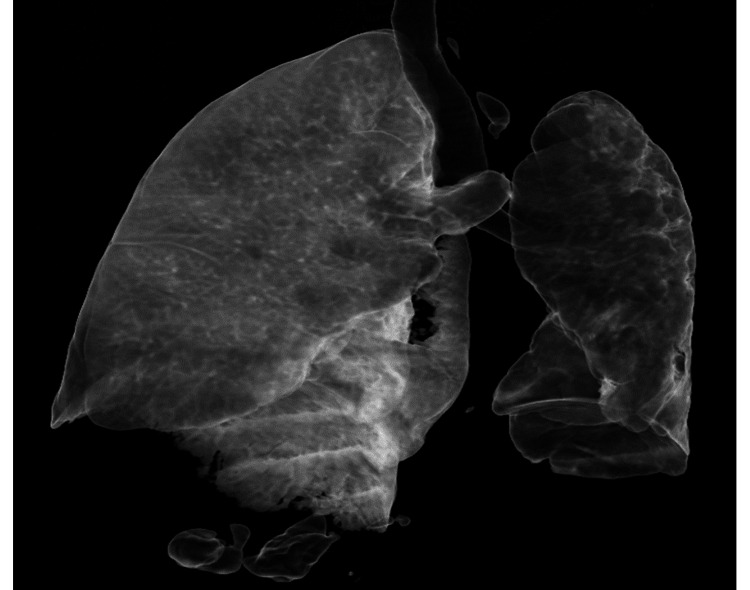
CECT chest 3D reconstruction image shows unilateral diffuse oligemia in the left lung. CECT: Contrast-enhanced computed tomography
